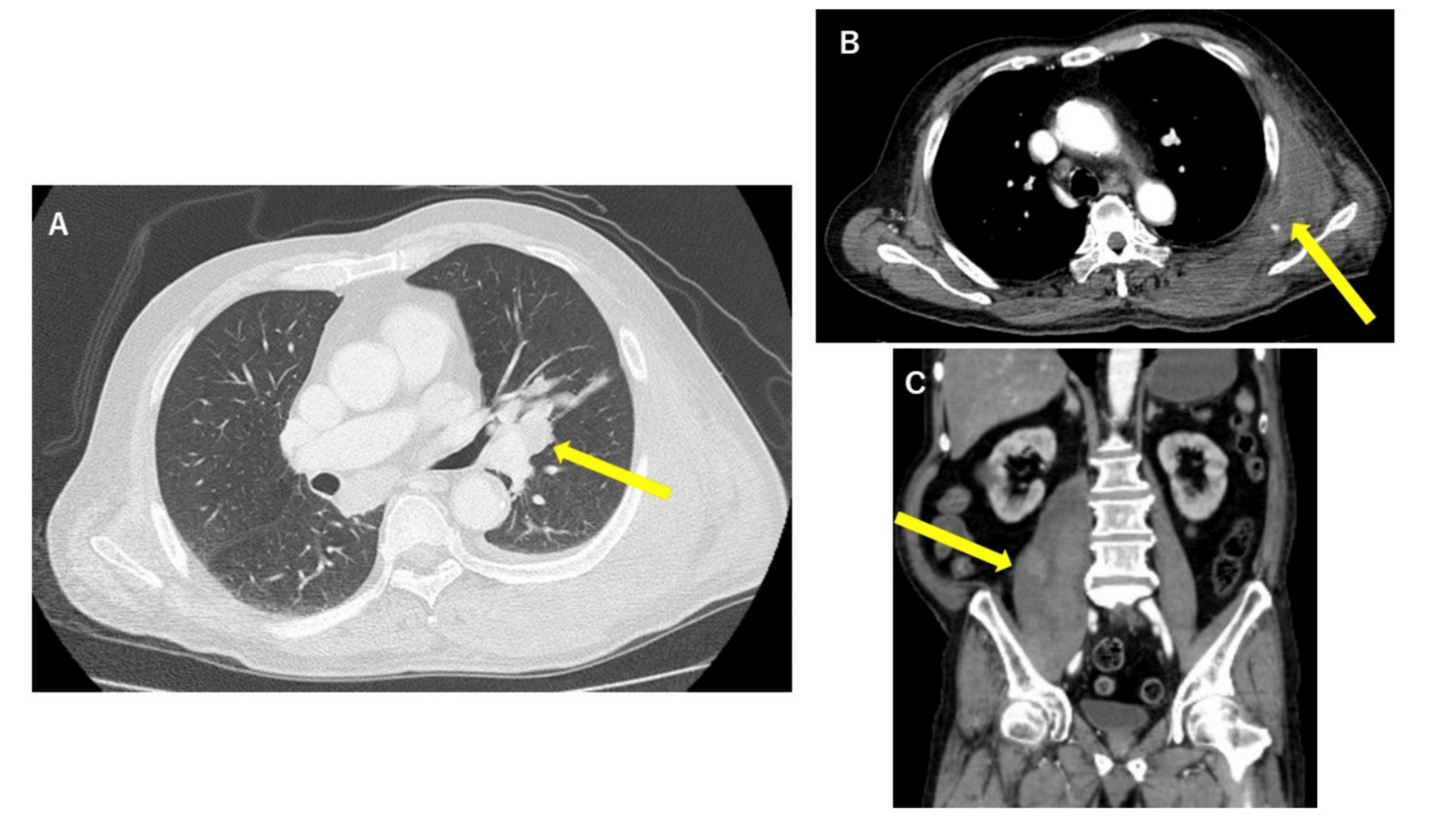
Contrast-enhanced CT of the thorax and abdomen A: Axial view, showing a 45 mm mass in the left pulmonary hilar region. B: Axial view, showing hematomas in the left back intramuscularly and subcutaneously. C: Coronal view, showing a hematoma in the right iliopsoas muscle.
